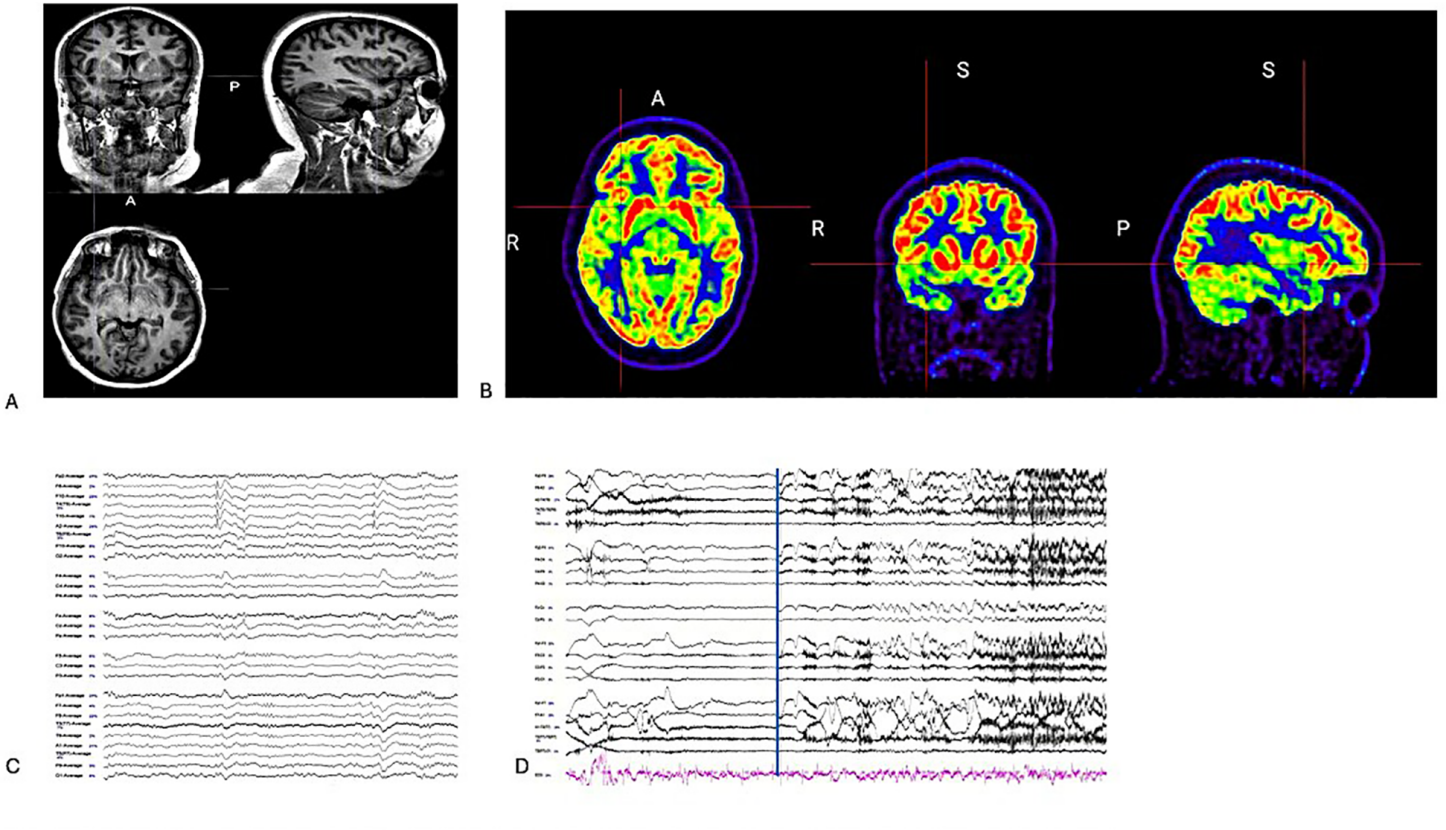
(A) Coronal/sagittal and axial T1 weight MRI. (B) Coronal/Sagittal and Axial PET-co-registered MRI showed hypometabolism in the right insula cortex. (C) On average montage, there are interictal discharges in the right temporal region during sleep stage 2. (D) On bipolar montage, the EEG seizure is not localized but lateralized to the right hemisphere during VEEG monitoring (The blue line was clinical seizure onset).
